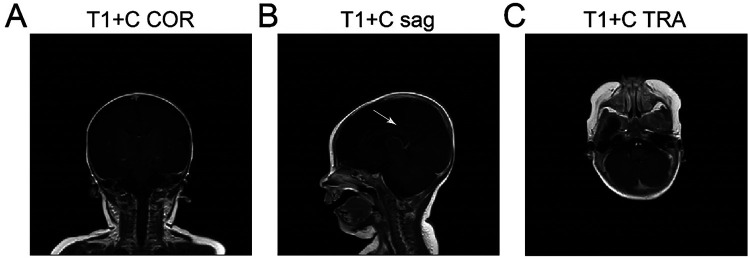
Contrast-enhanced brain MRI on march 27, 2025. (A) Coronal T1-weighted postcontrast image. (B) Sagittal T1-weighted postcontrast image. (C) Axial T1-weighted postcontrast image.
